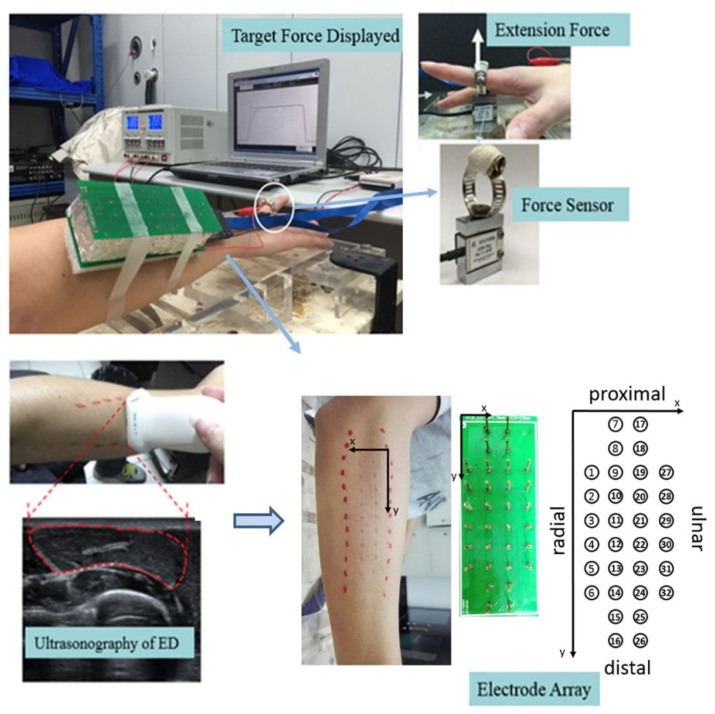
The scene of the experiment for sustained force-tracking through right index finger extension. The extension force of index was detected with a custom-made ring-like force sensor, and the sEMG was recorded with 32-channel circular electrodes of test probes. The edge of superficial region of ED muscle was identified using ultrasonography.
